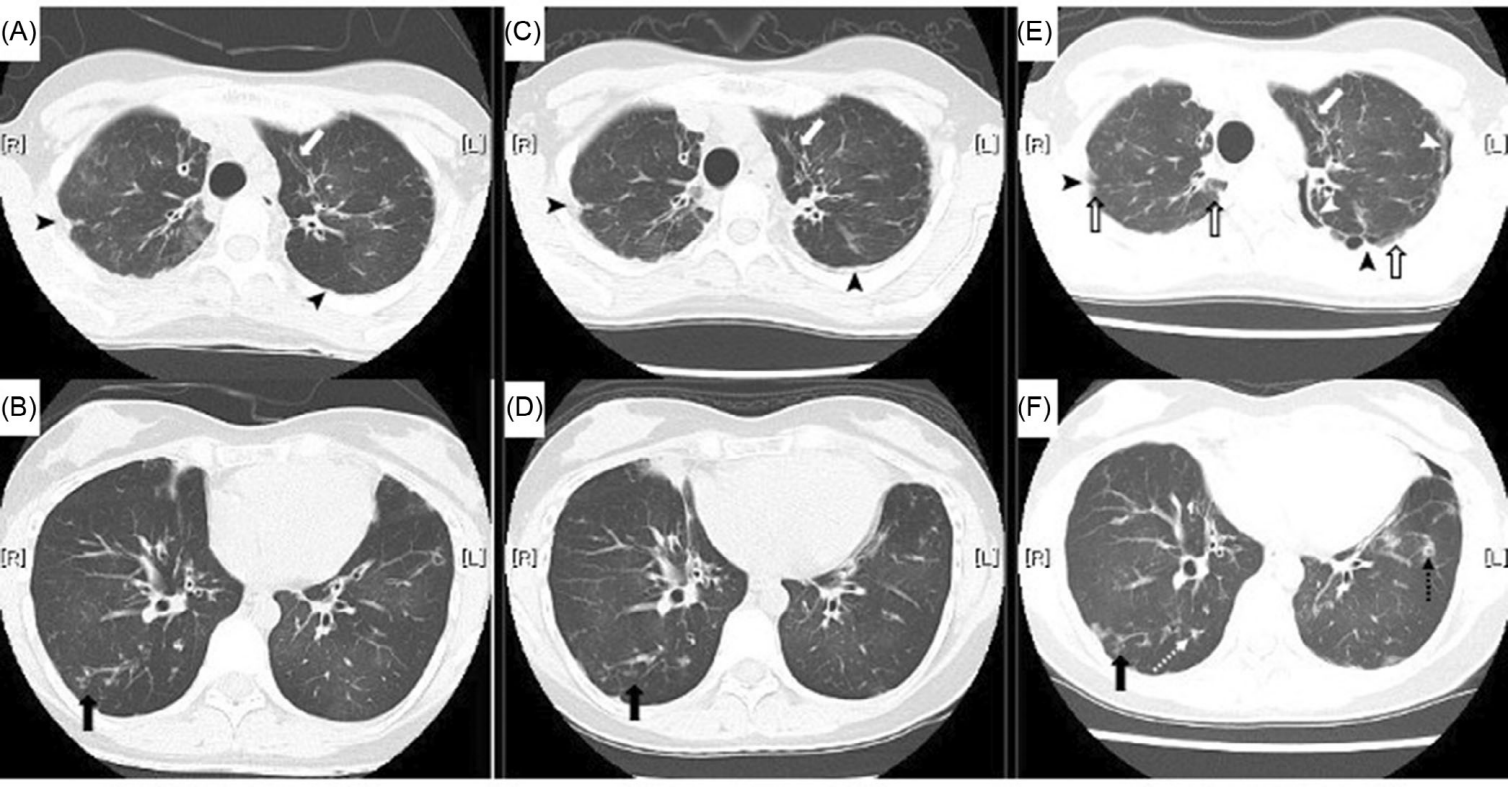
Computed tomography of the chest reveals a centrilobular granular shadow (black arrow), bronchiectasis (white arrow), and pleural thickening (black arrowhead) of the apex of both lungs at 3 years before presentation (A, B), slowly progressive bronchiectasis and pleural thickening at 2 years before presentation (C, D), and interstitial pneumonia of the upper lobe of both lungs (open arrow), pneumothorax (white arrowhead) in the left lung, bronchiectasis, bronchial wall thickening (dashed black arrow), and nodules (dashed white arrow) in both lungs (E, F).
